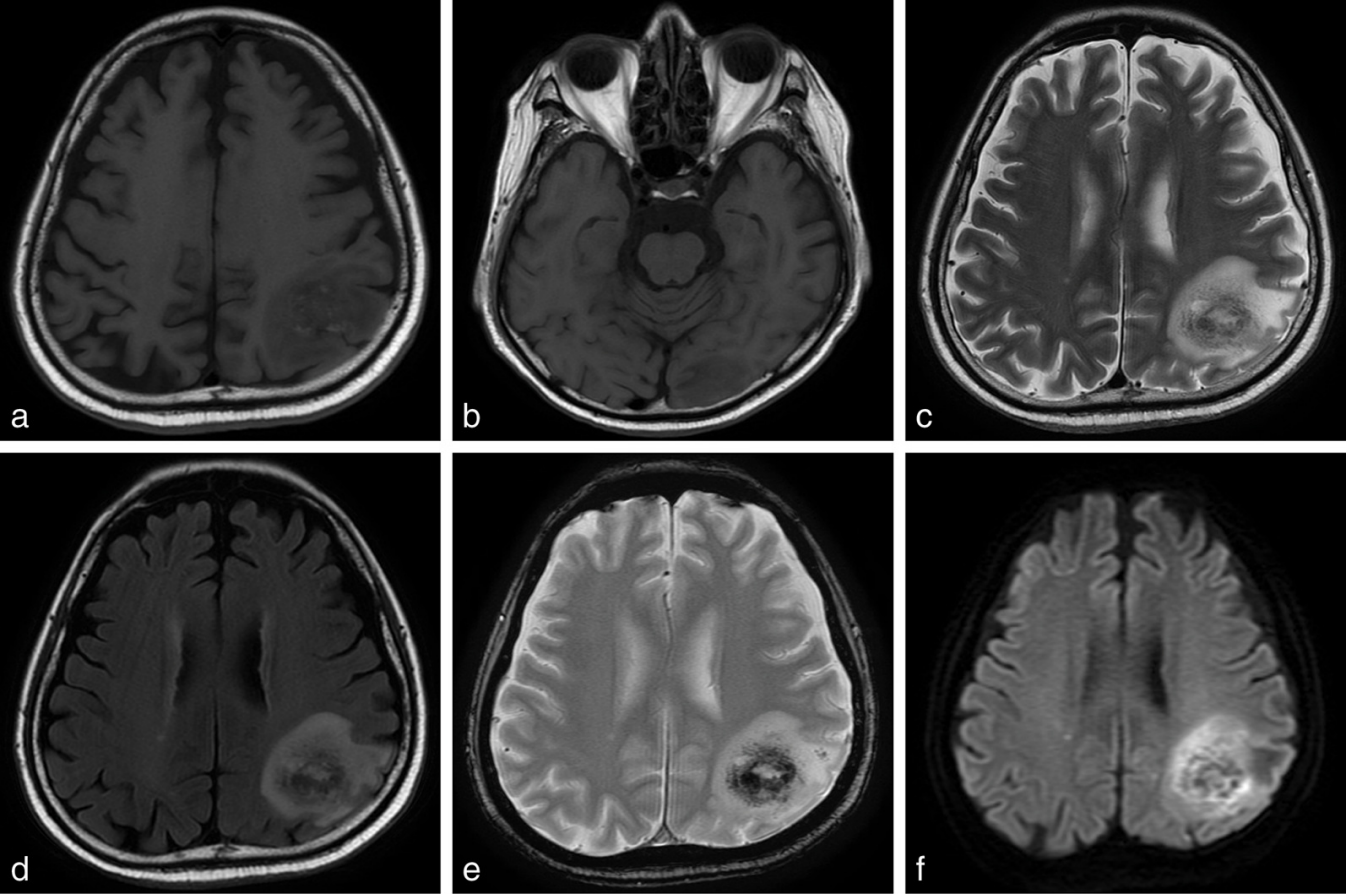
MRI of brain *T*_1_ (a, b), *T*_2_ (c), fluid-attenuated inversion recovery (d), *T*_2_ gradient echo (e) and diffusion-weighted images (f).
